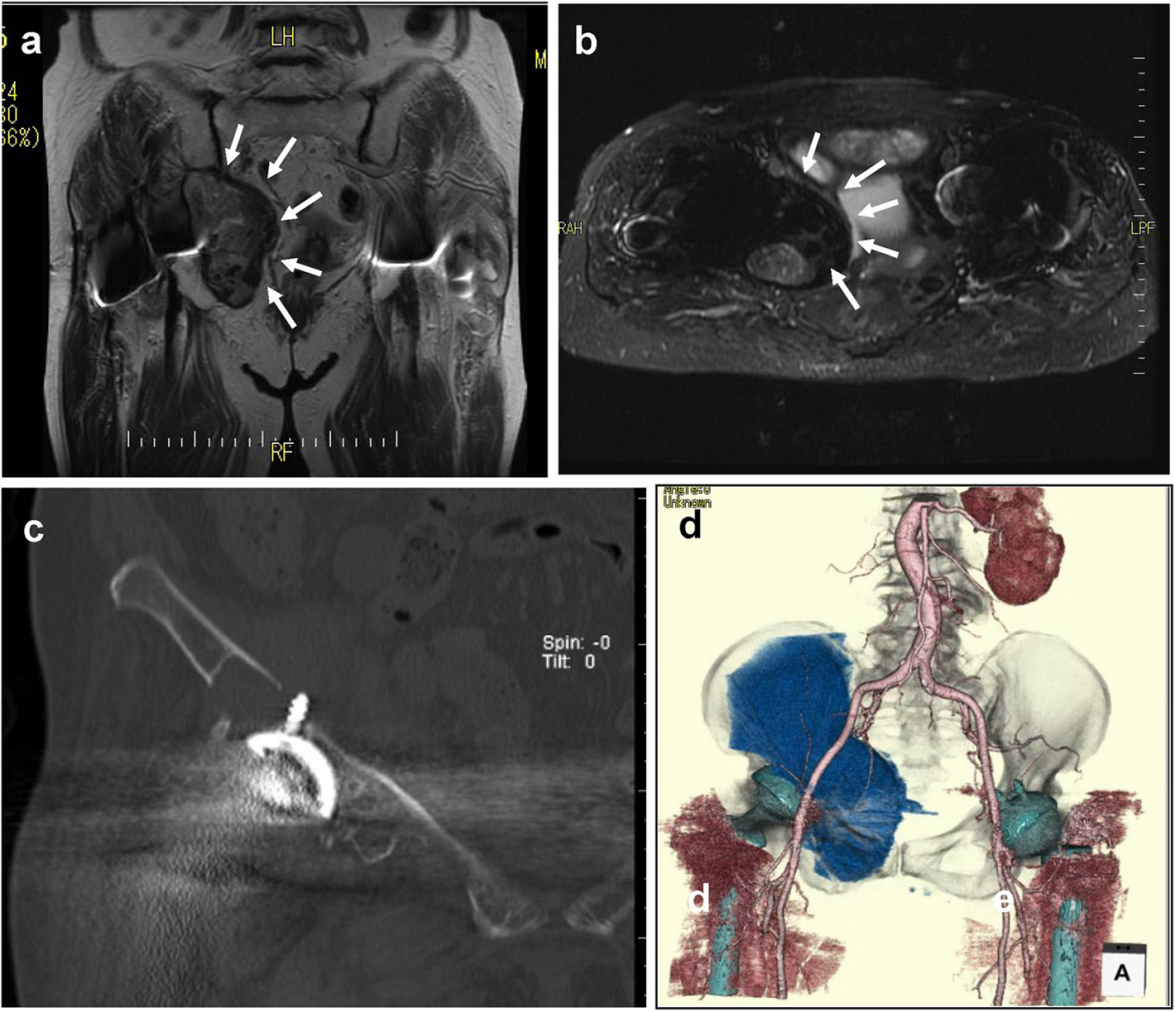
Imaging findings on admission. (a) A coronal T2-weighted magnetic resonance image (repetition time [TR] of 3800 and echo time [TE] of 74,932) shows a mass of low signal intensity within the pelvis (area surrounded by arrows). (b) A short T1 inversion recovery (STIR) image of magnetic resonance imaging (TR of 3700 and TE of 58,786) shows a small area of high density within the low-density lesion (surrounded by arrows). (c) High-resolution coronal computed tomography images show penetration of innominate acetabular bone (with partial bone resorption) by the screw tip and a large low-density lesion. (d) Three-dimensional magnetic resonance angiography shows a large abnormal lesion that is located between the external and internal artery and vein and that extends widely to the obturator foramen or foramen obturatum.
